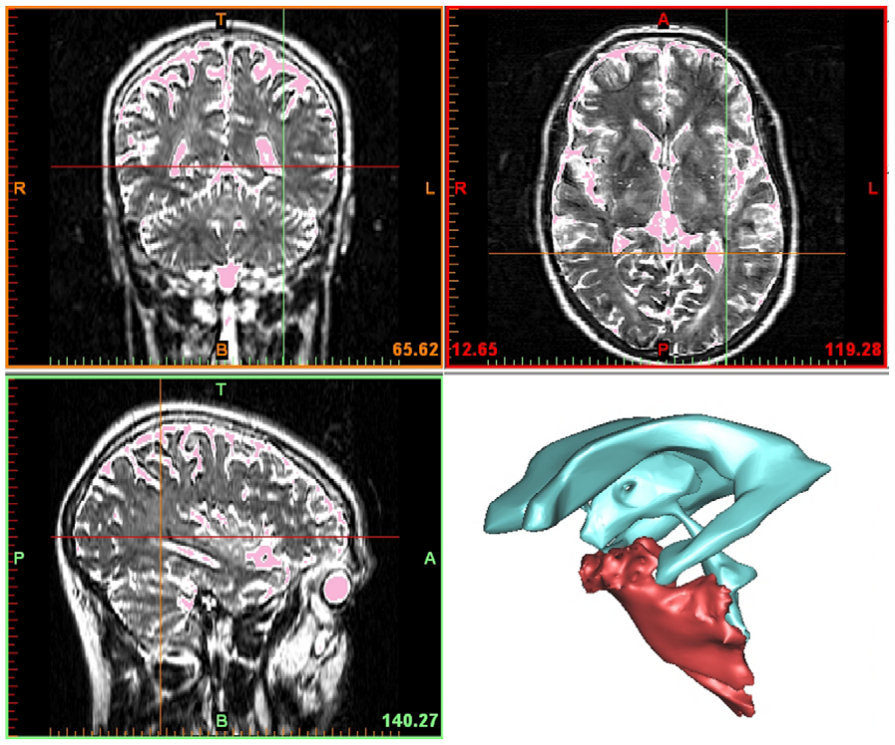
Anatomical data of the model cistern. Three orthogonal slices of the MRI data with cerebrospinal fluid in the cerebral sulci, ventricles, and basal cisterns indicated in pink. The three-dimensionally reconstructed basal cistern (red) and the ventricular system (blue) are shown for a better space orientation.
